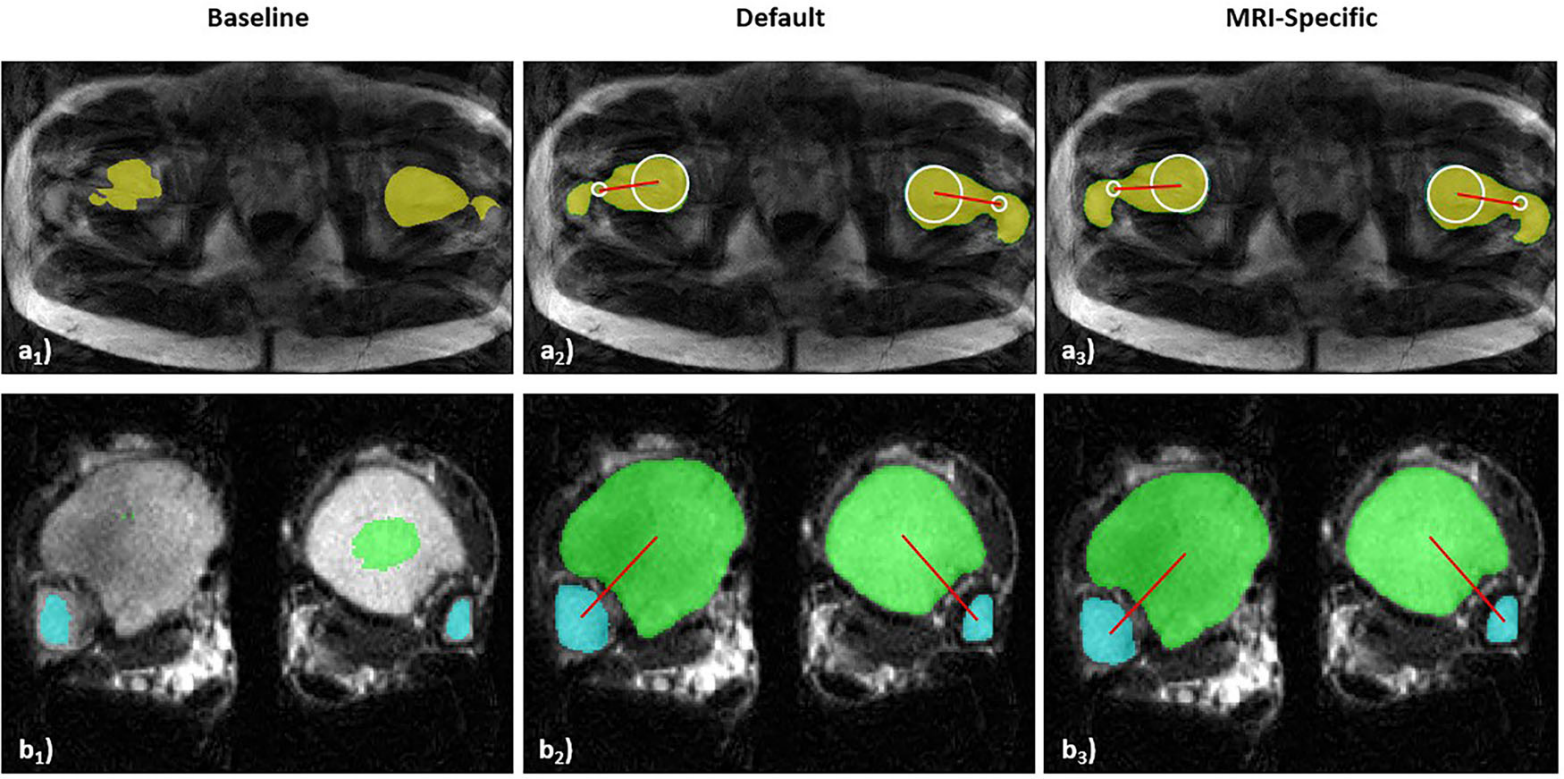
Examples of failed automatic torsion measurement. MR images of the proximal femur (a) and distal tibia (b) with a high grade of artifact degradation. Computed segmentation outlines and reference lines are overlaid. Color coding and image overlays as in Fig. 1. The quality of the segmentation outlines computed by the baseline model (a_1_ and b_1_) was too poor for the model to determine any reference lines. In contrast, the models enhanced with default (a_2_ and b_2_) and MRI-specific (a_3_ and b_3_) augmentation produced segmentation outlines of sufficient quality for determining reference lines. There were no instances in the test data where the model failed to determine the knee reference lines
